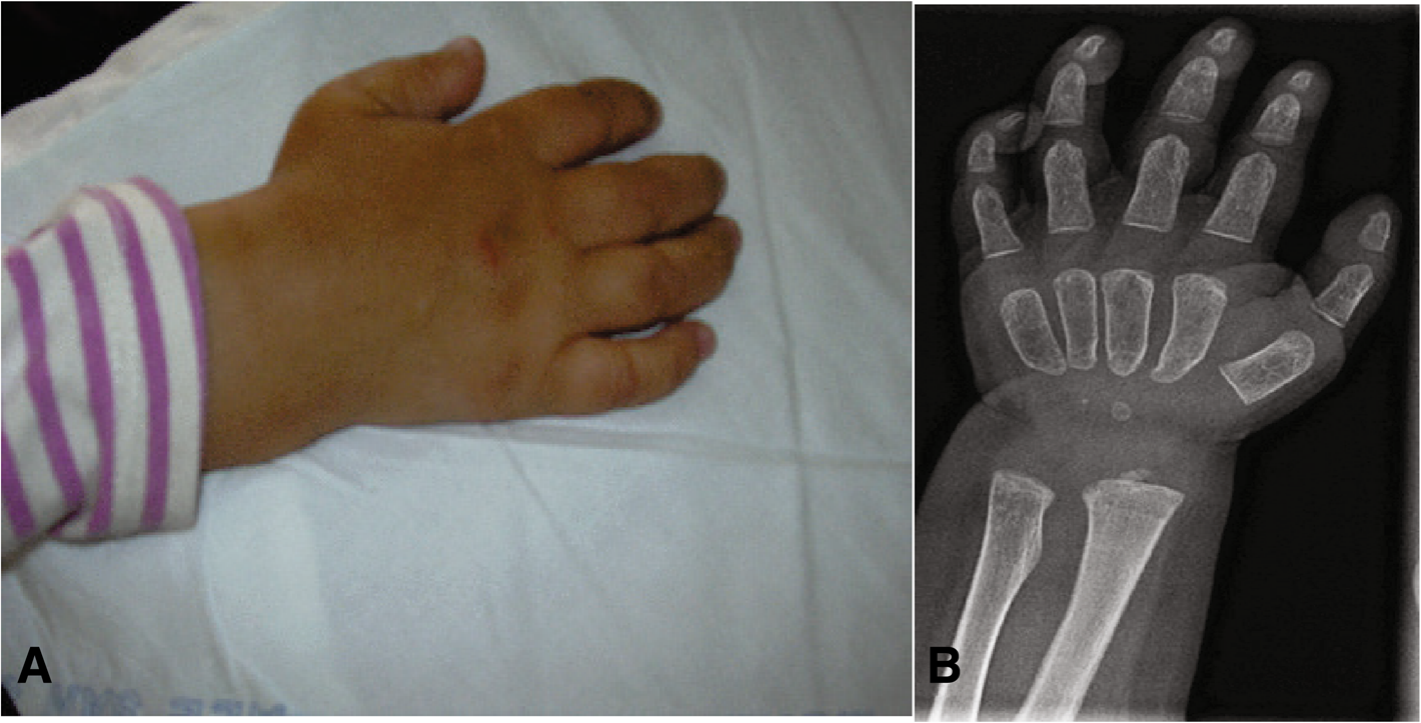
MPS IH: a Claw hand in an 18 month-old patient; b hand X-ray.
